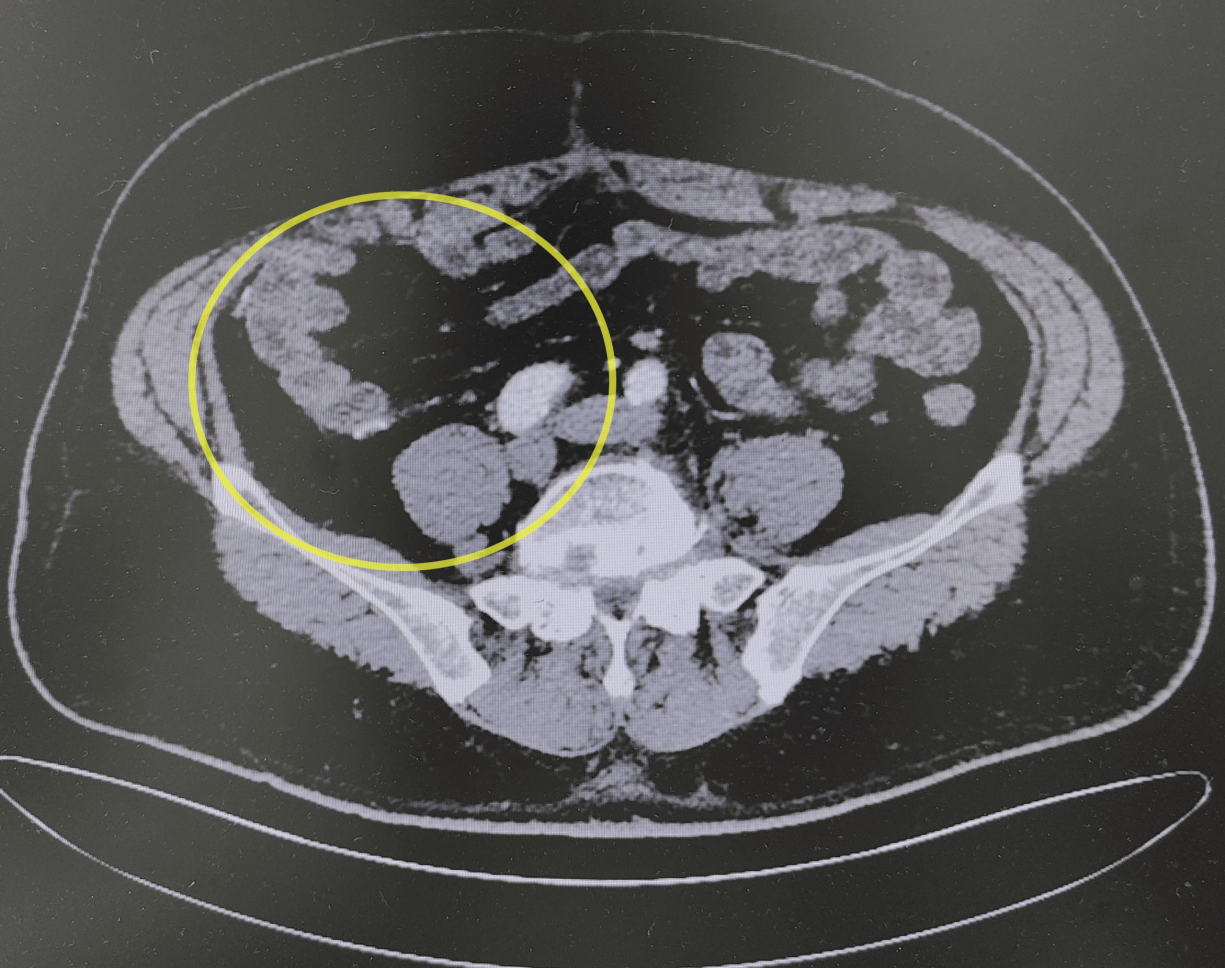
The follow-up CT of the abdomen showed postoperative changes in the right abdomen, with no recurrence or metastasis observed
